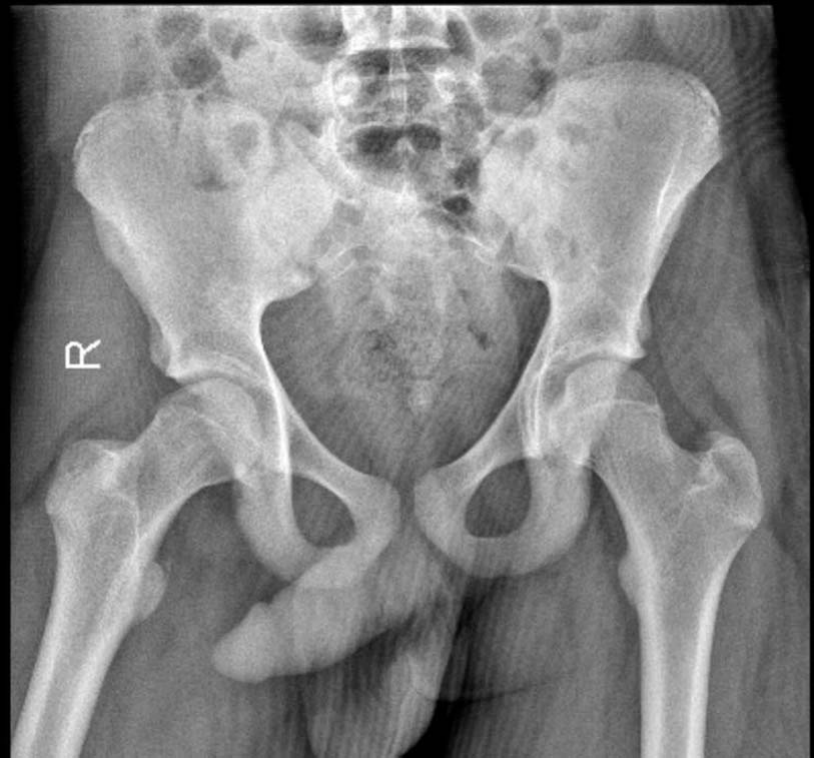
An anterior-posterior pelvic view X-ray after reduction. The femoral head is seen in the acetabulum.
